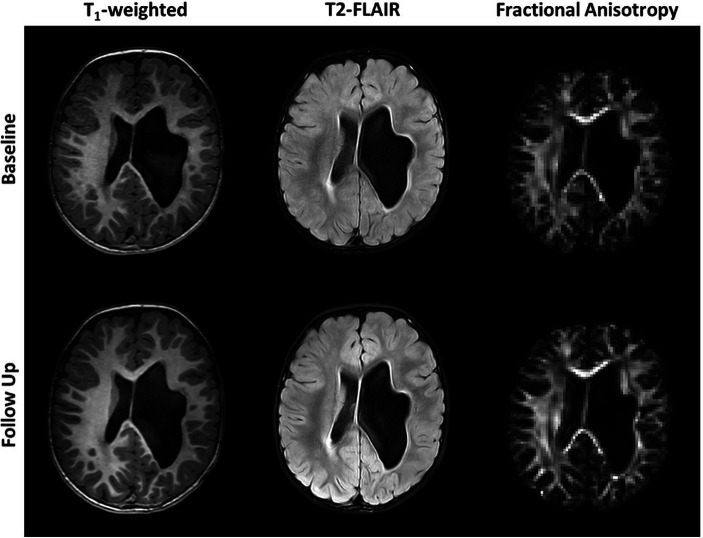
Axial unenhanced T1-weighted, T2-FLAIR images and fractional anisotropy (FA) maps of subject 5 before (Baseline) and after 36 weeks of P-AA physical therapy. No significant changes are visible in the images or maps. Subject 5 was chosen as an example and all other subjects showed identical results, where no significant changes are noticeable in the images and FA maps.
